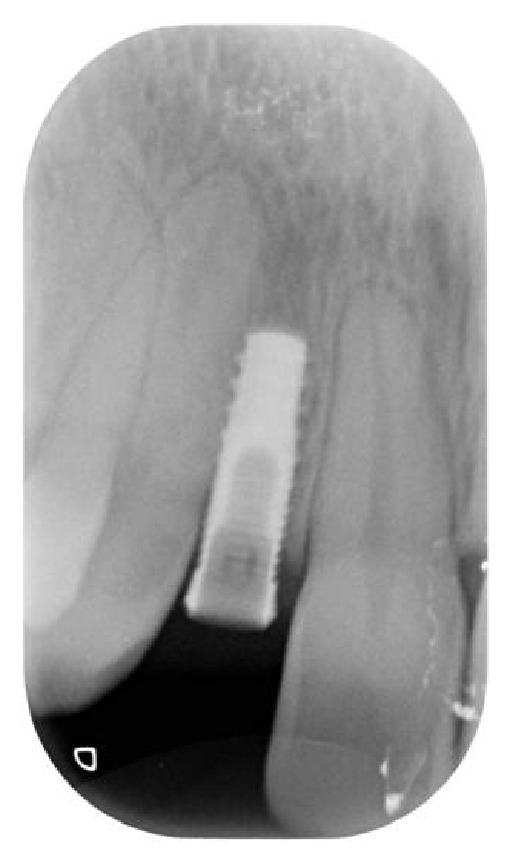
Radiographic control after implant placement in site to the left.
